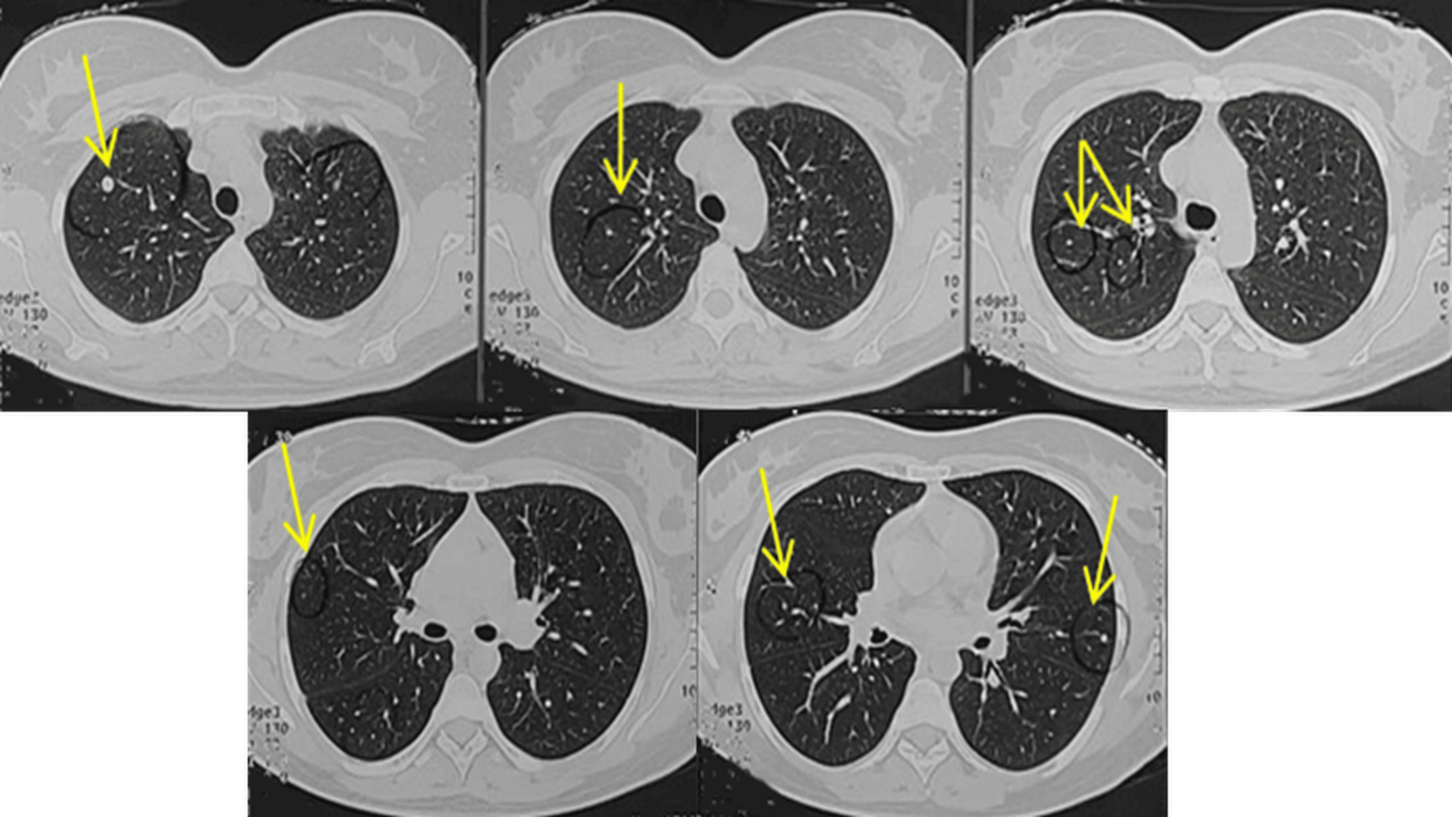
Axial chest CT images of the lungs obtained 23 years prior, showing multiple well-demarcated, round pulmonary nodules of varying sizes (yellow arrows)
